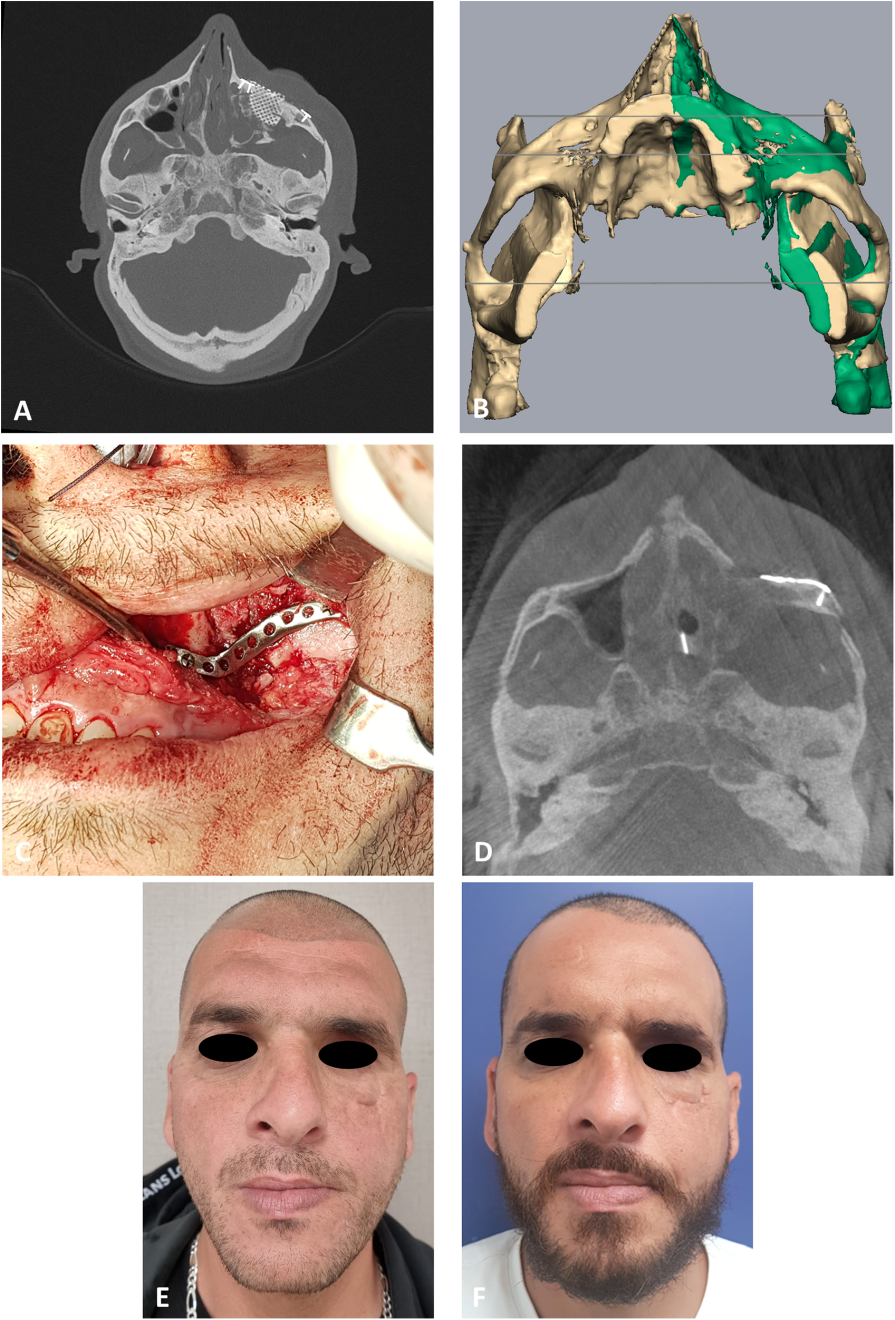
Second case presentation. (A) CT stacking* of a preoperative axial view demonstrating the depressed zygoma on the left following an unsuccessful surgical intervention. * Overlaying multiple CT scans into a 2D image. (B) Mirroring (green) of the right uninjured side to the left injured side. Mirror image is then used as a guide for the desired position of the left zygoma. (C) Intraoperative, intraoral view of the PSI fixating the refractured and repositioned left zygoma. (D) CT stacking of a intraoperative axial view demonstrating the leveled malar points following fixation with the PSI. (E) Clinical preoperative en face view following the first surgery demonstrating the depression of the left malar point. (F) Clinical postoperative en face view following the second 3D guided refracture and repositioning surgery. Notice the reconstructed malar point.
